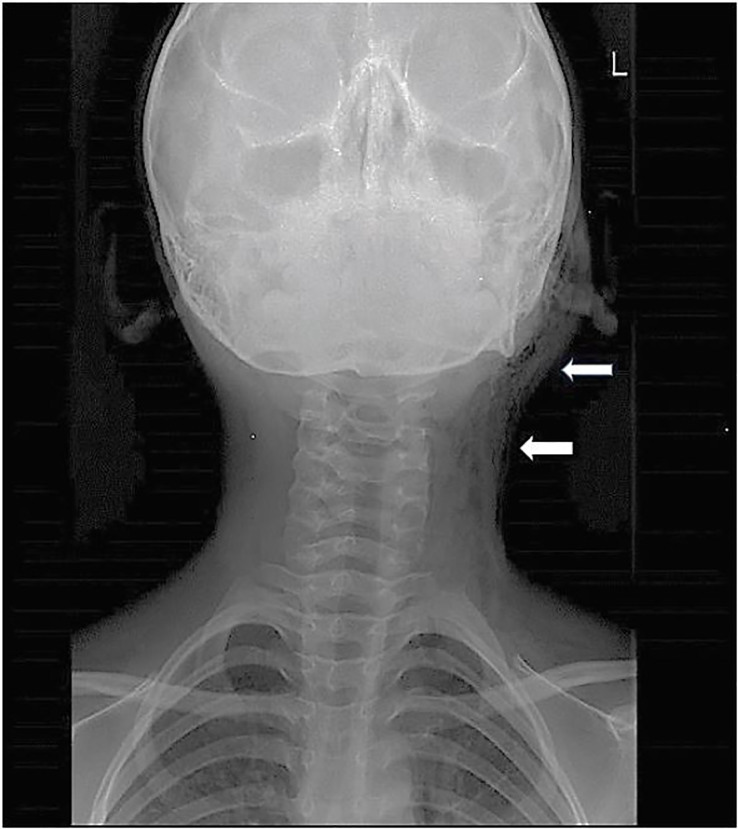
Anterior-posterior radiograph of the neck demonstrating extensive left-sided cervicofacial subcutaneous emphysema (arrows).
